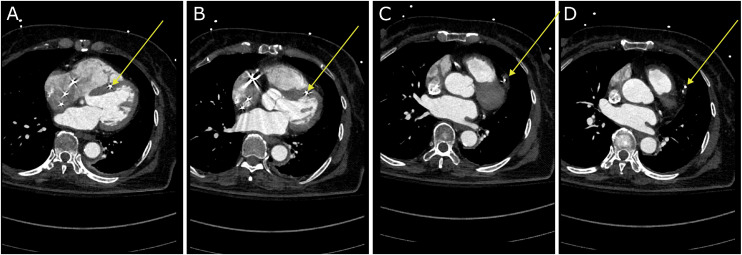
Gated CT angiogram of the chest, showing the right ventricular lead migration through the (A) and (B) interventricular septum towards the apex, (C) through the apex, and (D) into the left upper lobe (yellow arrow).
